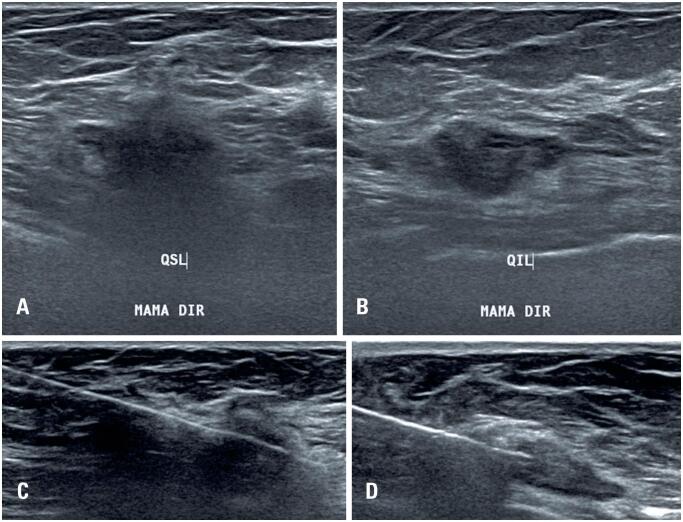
Ultrasound of the right breast showing two hypoechoic, irregular masses with spiculated margins, malignancy, located in the upper outer quadrant (A) and lower outer quadrant (B) Ultrasound-guided core needle biopsy was subsequently performed (C - upper outer quadrant, D - lower outer quadrant)
